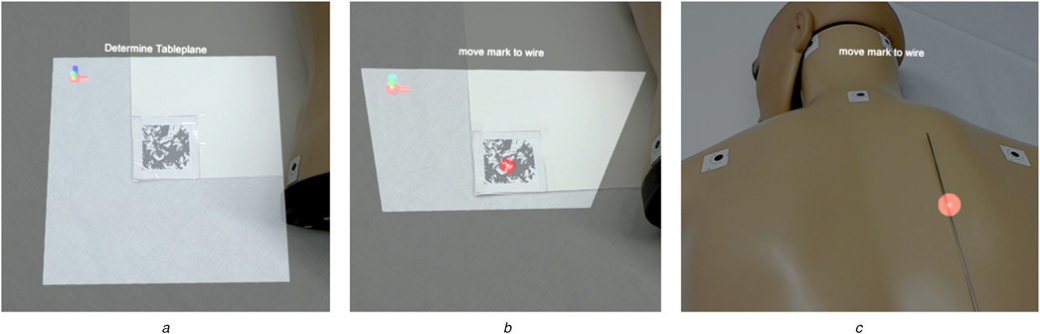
Image marker-based registration approach between the HoloLens and a desired world coordinate space, e.g. the CT scanner *a* Image marker tracking. A marker corresponding with the patient table is tracked using the *Vuforia AR SDK* *b* CT plane calculation. The CT plane, i.e. the plane corresponding with the CT coordinate system, is determined as the plane perpendicular to the tracked image marker *c* Position definition. A mark is manually moved to the respective scanning position
